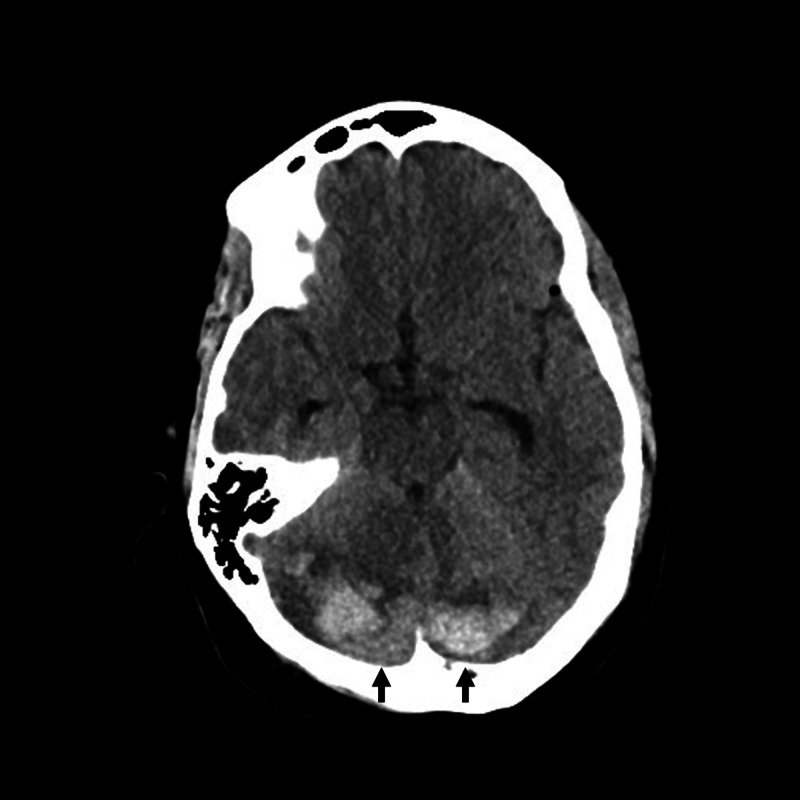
Axial CT indicating post-operative day one bilateral RCH in the superior cerebellum. RCH: remote cerebellar hemorrhage
